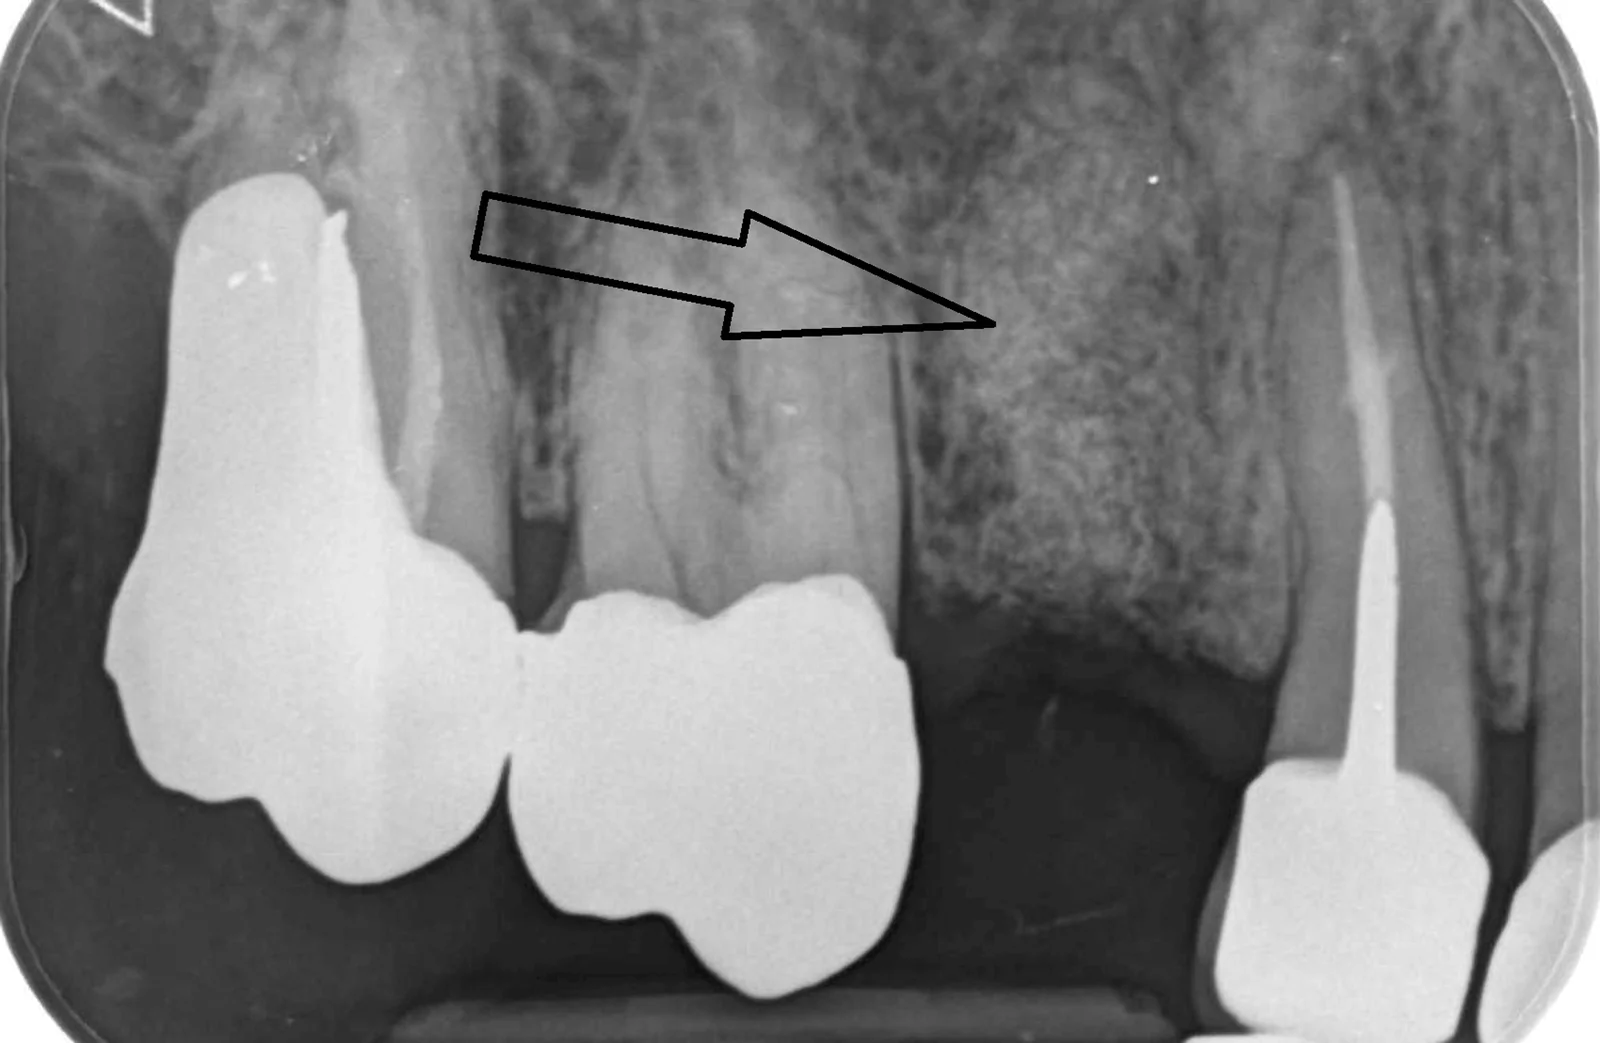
Post-extraction periapical radiograph demonstrating alveolar ridge preservation of the maxillary right second premolar (tooth #4). The black arrow indicates the particulate bone graft filling the extraction socket, which was covered with a resorbable collagen membrane to preserve alveolar ridge dimensions for future implant placement.
